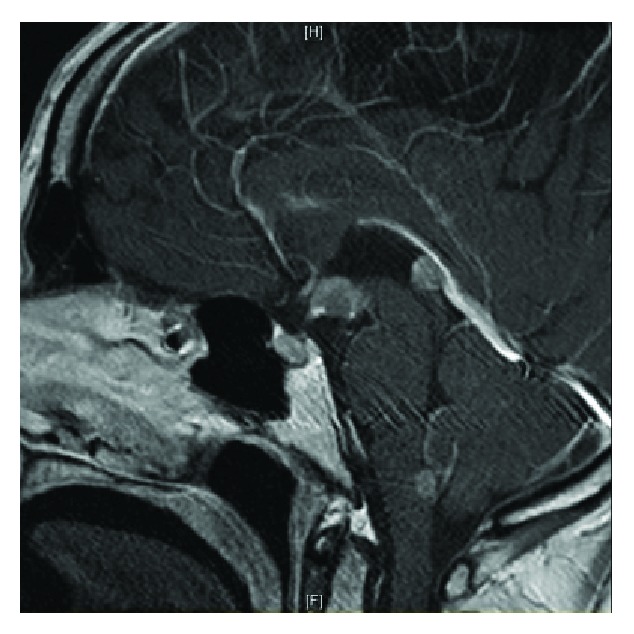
MRI brain, T1 sagittal + gadolinium, demonstrated lesions within hypothalamus, pineal gland, trigon of the right lateral ventricle, and foramen of Magendie at diagnosis.
